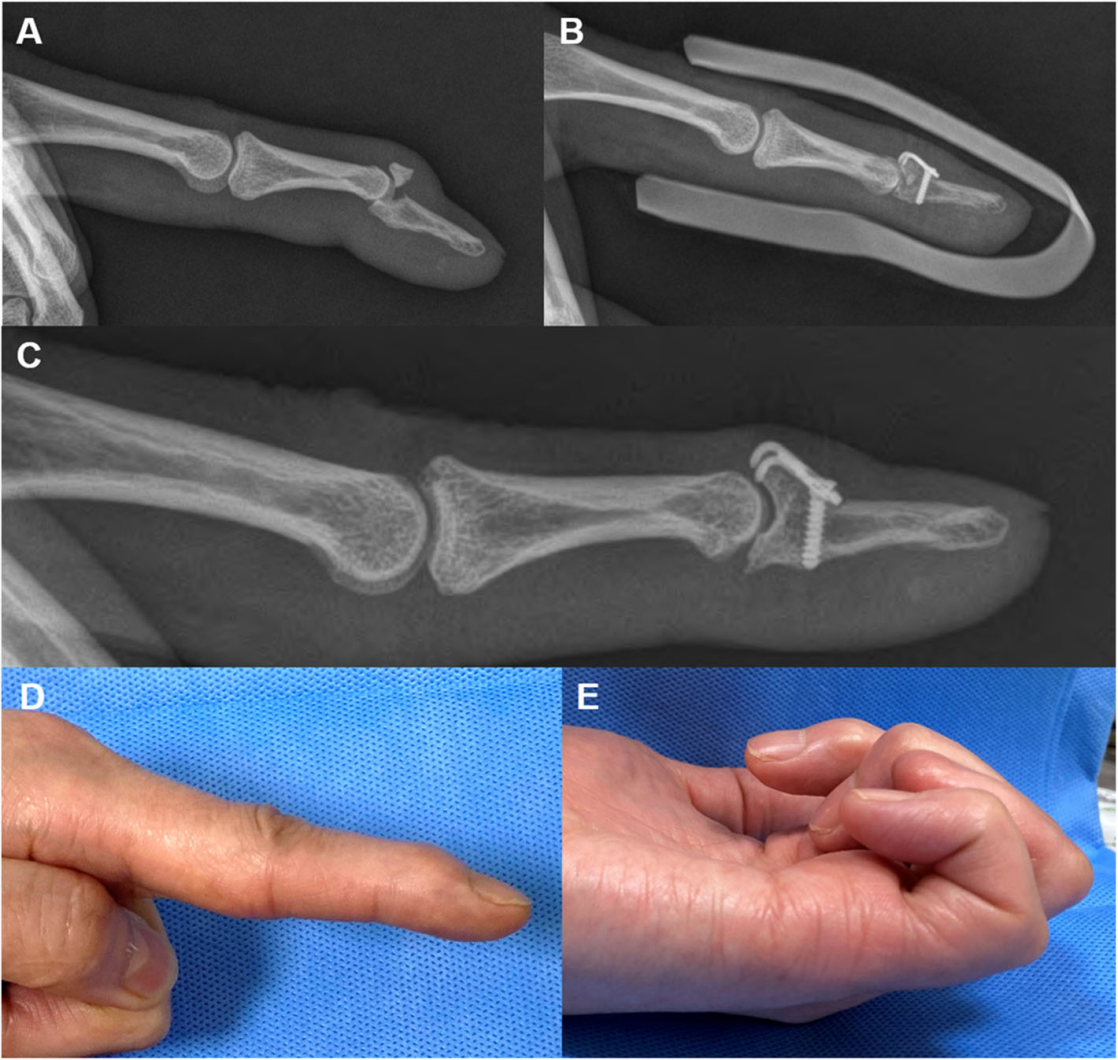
Mallet fracture (a). The mini-hook plate captured the dorsal avulsed fragment, and the joint was reduced (b). Plain radiography 5 years post-operation revealing that osseous union, no metal failure, and no subluxation of the joint. However, an irregular articular surface is visible (c). The total range of DIP joint motion was 69%, and the pinching strength was 43% compared to the values of the same contralateral joint (d, e)
